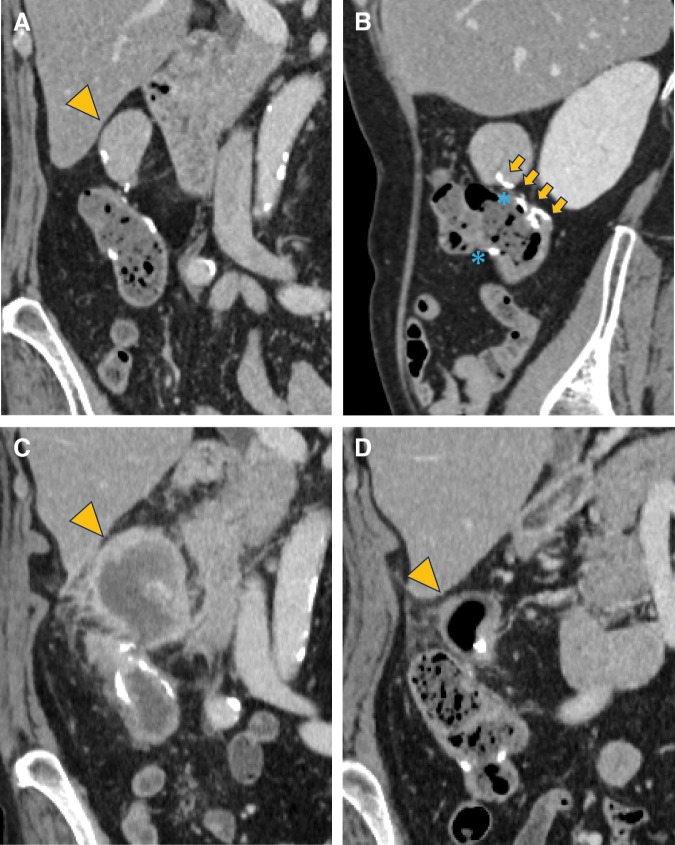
Contrast-enhanced CT findings. (A) Five years after surgery, before inflammation onset: A 35-mm dumbbell-shaped low attenuation mass protruding extramurally from the FEEA stapler line (arrowhead). The mass had a smooth surface, homogeneous internal contents, and no contrast enhancement (coronal view). (B) The cyst appears to be contiguous with the stapler line of the stump. The stapler line of the common orifice (asterisk) and that of the stump (arrows) are indicated (sagittal views). (C) At the time of inflammatory onset, 6 years and 6 months postoperatively: The mass (arrowhead) has enlarged to 44 mm, with wall thickening and increased surrounding fat stranding. No solid internal components, wall disruption, or perilesional fluid collection suggestive of rupture is observed. (D) After conservative treatment: The mass (arrowhead) has decreased to 35 mm, with wall thickening and adjacent fat stranding inflammation. Air is newly visible within the cyst. FEEA, functional end-to-end anastomosis
